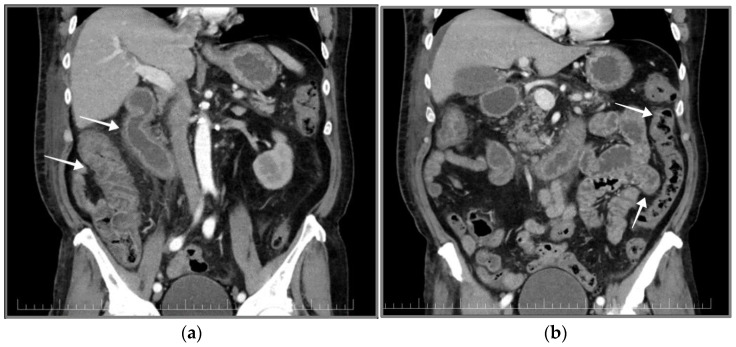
Contrast CT scan of the thorax, abdomen and pelvic region. (a) Ascending colon and duodenum with contrast-enhanced thickened wall (white arrows); (b) small bowel and descending colon with normal aspect (white arrows).
